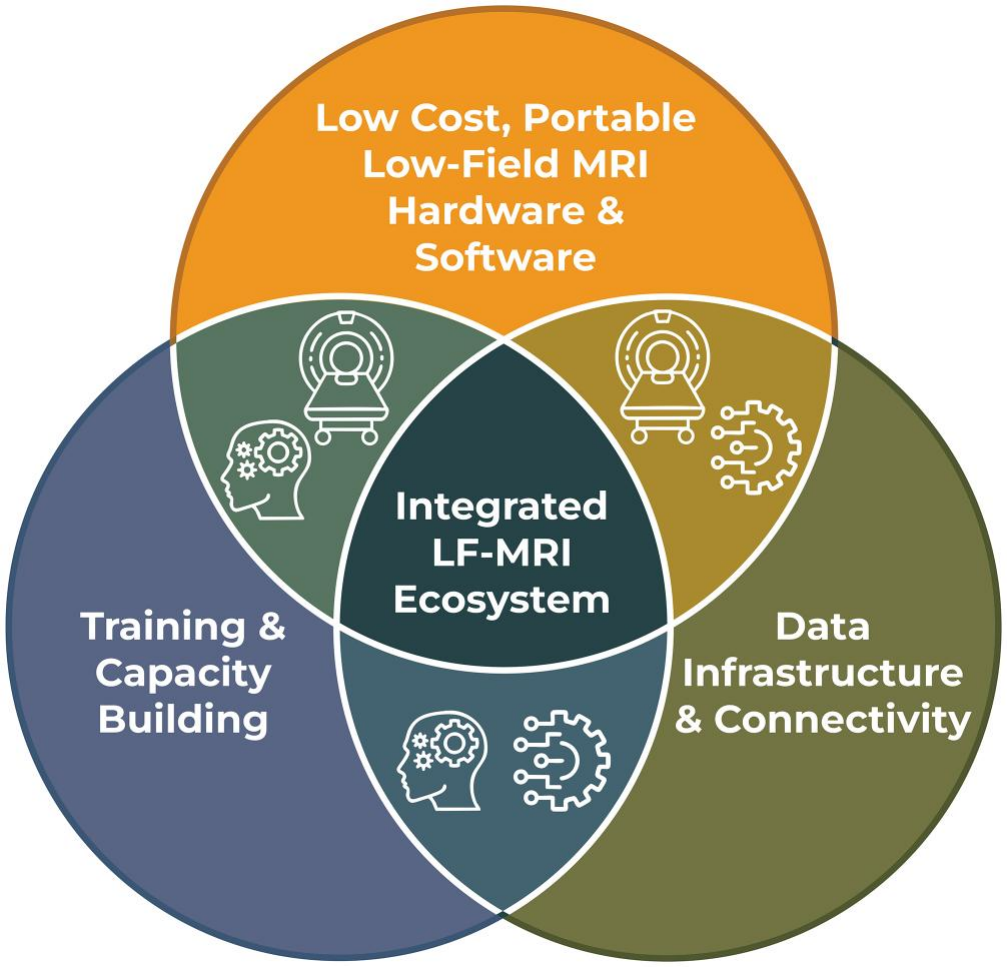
Integrated LF-MRI ecosystem: *People*, *Platforms*, and *Partnerships*. The foundational pillars required for the scalable and equitable deployment of LF-MRI in LMICs. *People*: Focuses on empowering local leadership and innovation. It emphasizes LF-MRI as a frontier for discovery tailored to LMIC health needs, fostering global networks, and building capacity through open-access training, mentorship, and inclusive representation in scientific governance. *Platforms*: Encompasses the design of sustainable, open-source MRI systems with modular hardware, accessible data. It includes infrastructure for ethical data governance, scalable storage and analysis, and regional engineering hubs to support maintenance and innovation. *Partnerships*: Advocates for inclusive collaboration between LMIC institutions, industry, and philanthropic organizations. It calls for co-development of certified systems, investment in scalable deployment, and recognition of LF-MRI’s role beyond clinical settings. Abbreviations: LF-MRI = low-field MRI; LMICs = low- and middle-income countries.
